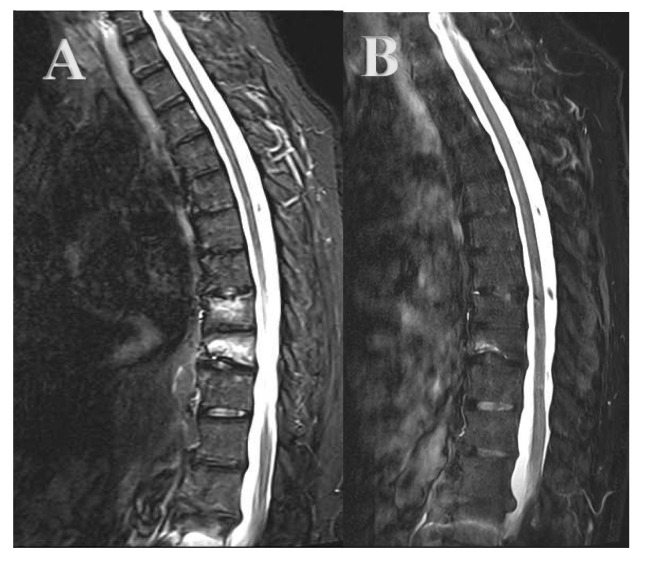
76 y.o. patient with T8 and T9 vertebral body fracture. (A) Sagittal short tau inversion recovery (STIR) MR images showing MES 4 at the T9 level and MES 3 at the T8 level. (B) STIR MR images after 2 months of conservative treatment and oral food supplement treatment, showing MES 1 at T8 and T9 vertebral bodies.
